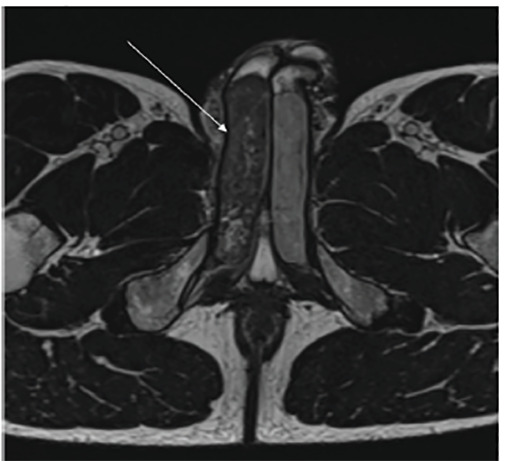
Axial T2-weighted magnetic resonance imaging of the pelvis revealing a hypointense signal of the right corpus cavernosum.
